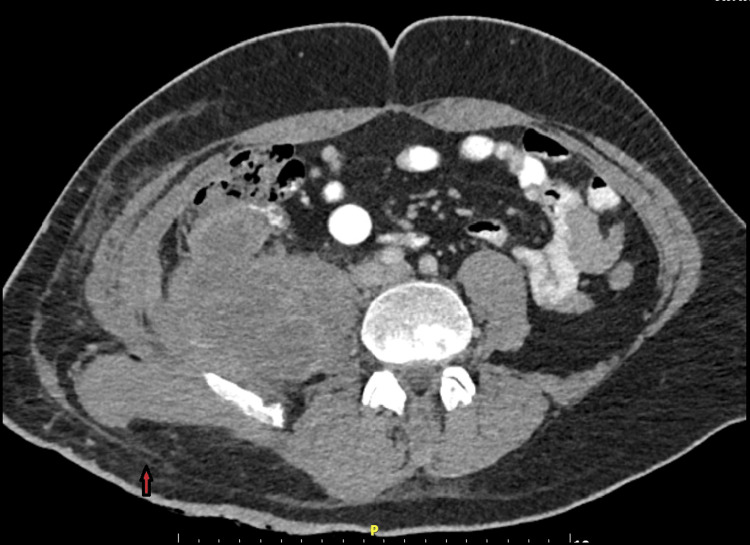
CT findings of follow-up examination revealing the abscess drainage area (red arrow).
